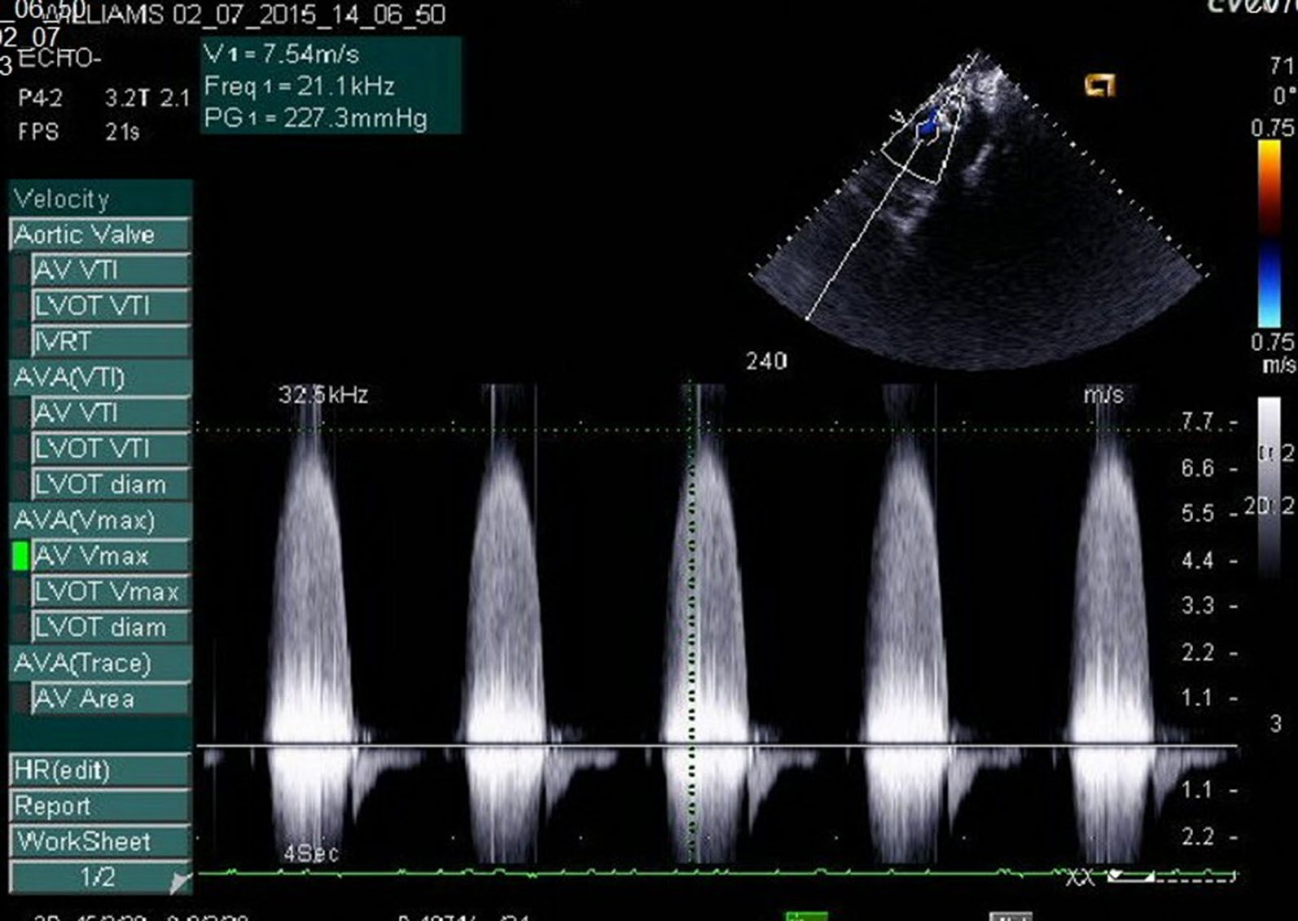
Continuous wave Doppler echocardiography in suprasternal view showing supravalvular aortic stenosis with peak systolic gradient of 227.3 mmHg
